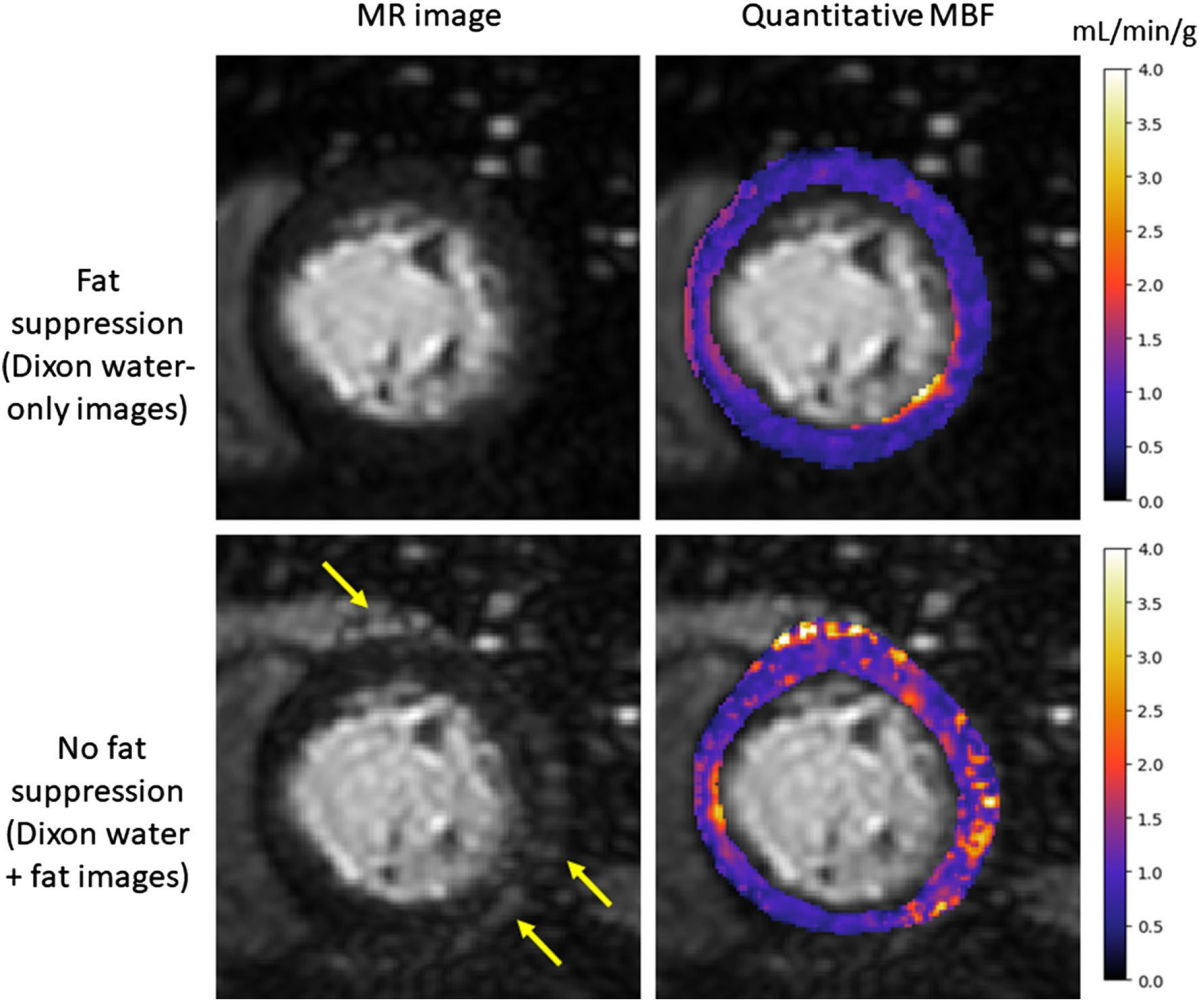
The MR images and corresponding quantitative MBF maps for a representative patient computed with both the water-only and the water and fat images. The benefit of the fat suppression is evident in the water and fat images. The fat, which appears as areas of high signal intensity (yellow arrows), leads to corresponding areas of spurious MBF values that are not present on the water-only images.
